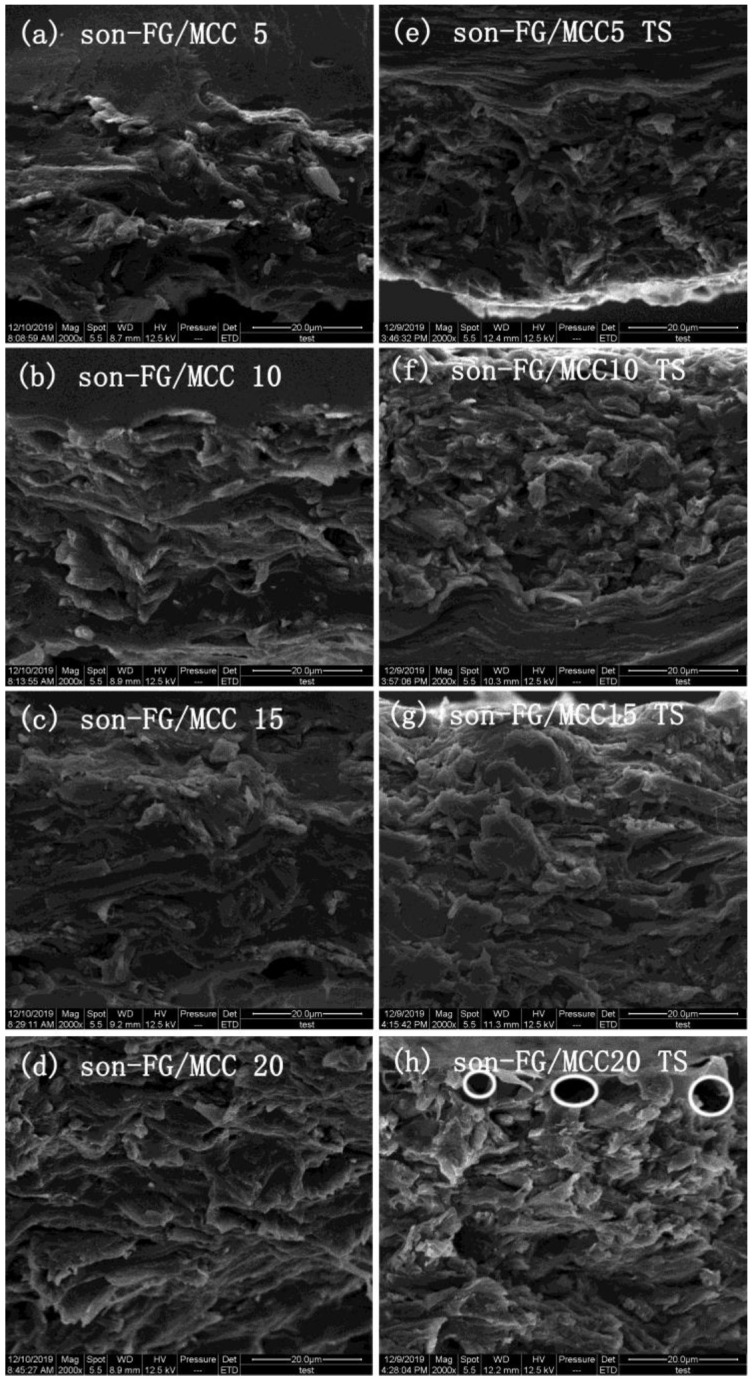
SEM images of the liquid nitrogen brittle section and tensile section (TS) of (a) son-FG/MCC 5, (b) son-FG/MCC 10, (c) son-FG/MCC 15, (d) son-FG/MCC 20, (e) son-FG/MCC 5 TS (f) son-FG/MCC 10 TS, (g) son-FG/MCC 15 TS and (h) son-FG/MCC 20 TS treated by ultrasound.
